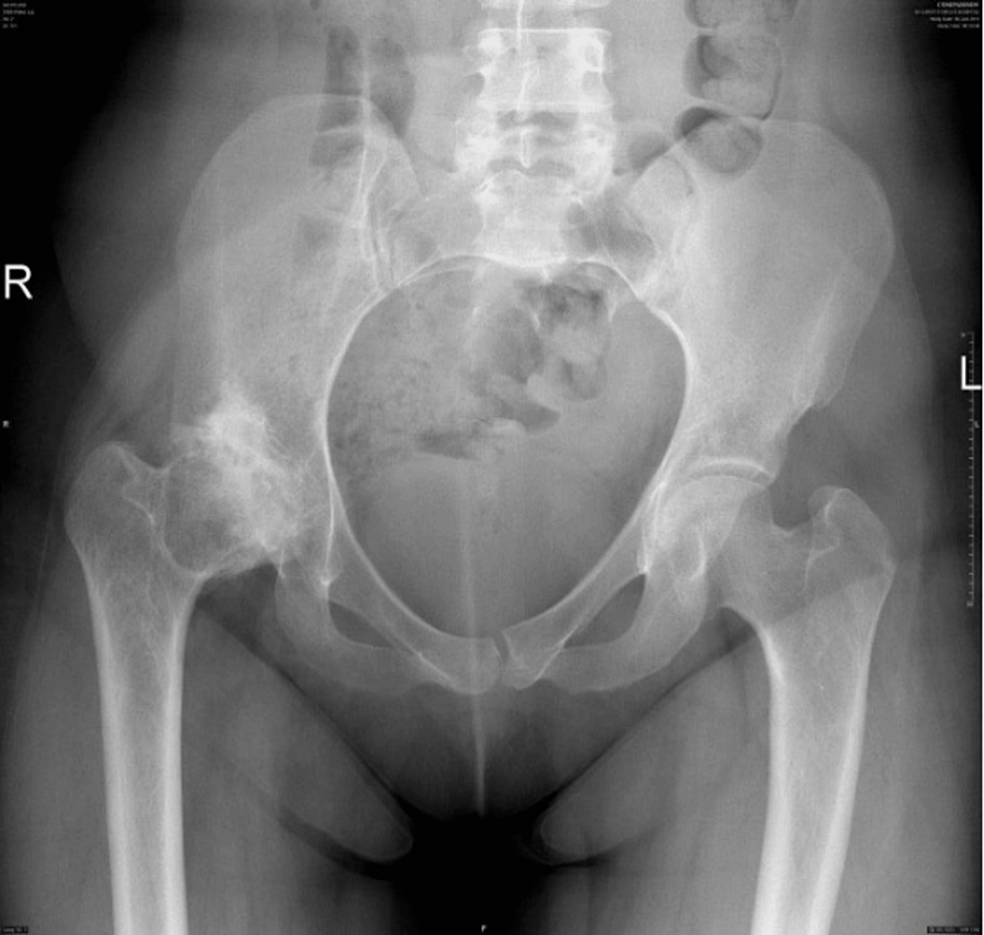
AP X-ray showing hip at the time of presentation. AP: Anteroposterior.
